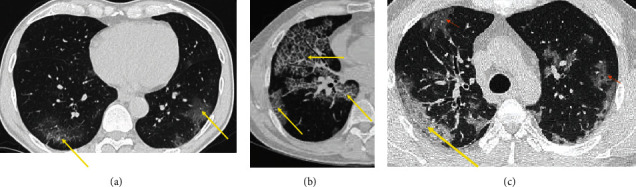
Chest CT lesions: (a) ground glass, (b) crazy paving, and (c) consolidation.
